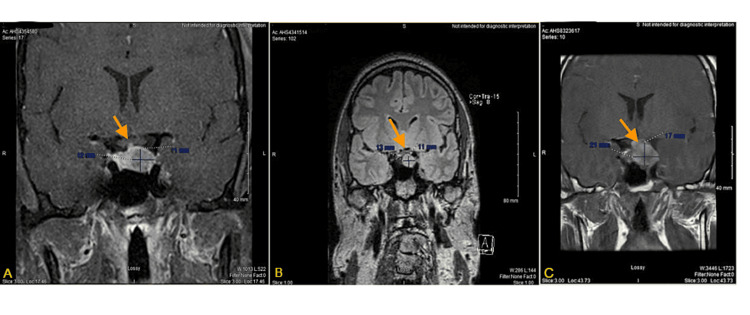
Progression of the tumor over three years from initial diagnosis: 12 x 11 mm (A) to six months after diagnosis: 13 x 11 mm (B) to just following presumed apoplexy event: 21 x 17 mm (C). The optic chiasm became compressed in second follow-up MRI (arrow in B).
